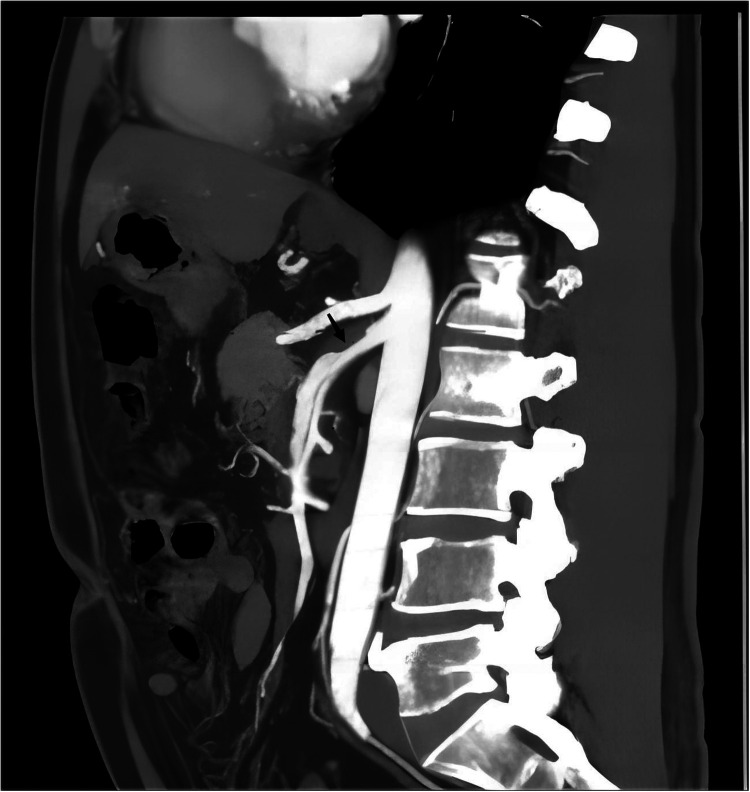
The typical performance of ISMAD in the sagittal plane of a computed tomography scan, with the black arrow pointing to the location of the dissection.
